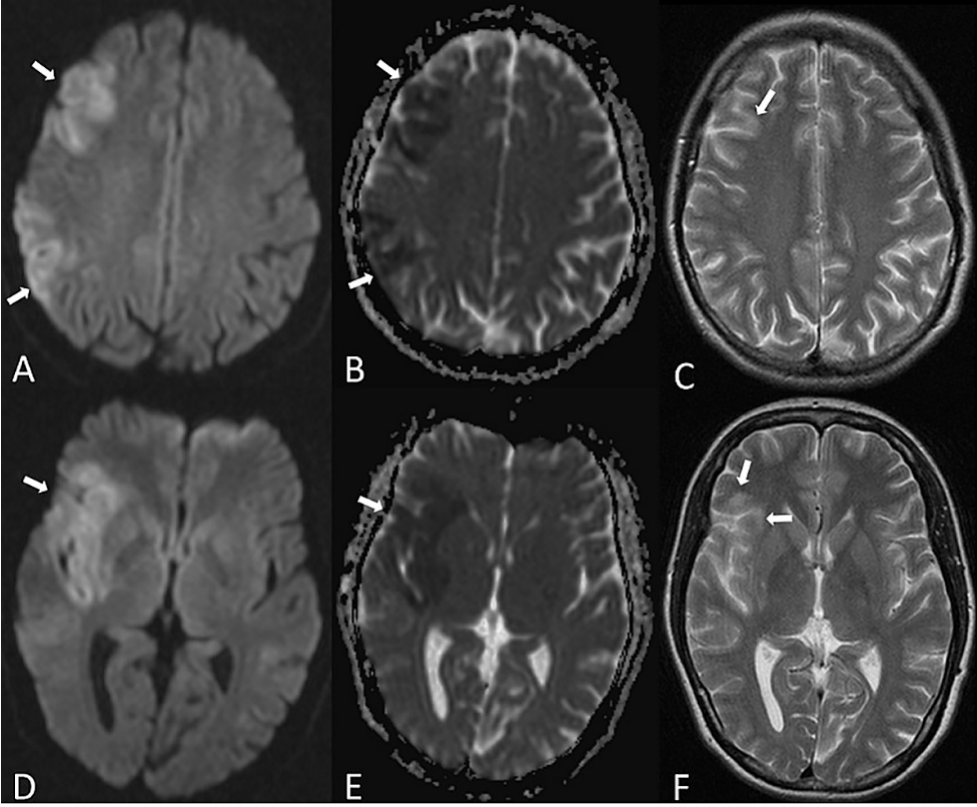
Typical pattern of a poorly-collateralized patient. 39y old female with right-sided M1-occlusion and a poor collateralization grade (Higashida grade 1); DWI (A,D), ADC maps (B,E) and T2w images (C,F). Diffusion restriction (A, B, D and E) is detected in the lentiform nucleus, insula, M1, M2, M4, M5 and M6 according to ASPECTS.
